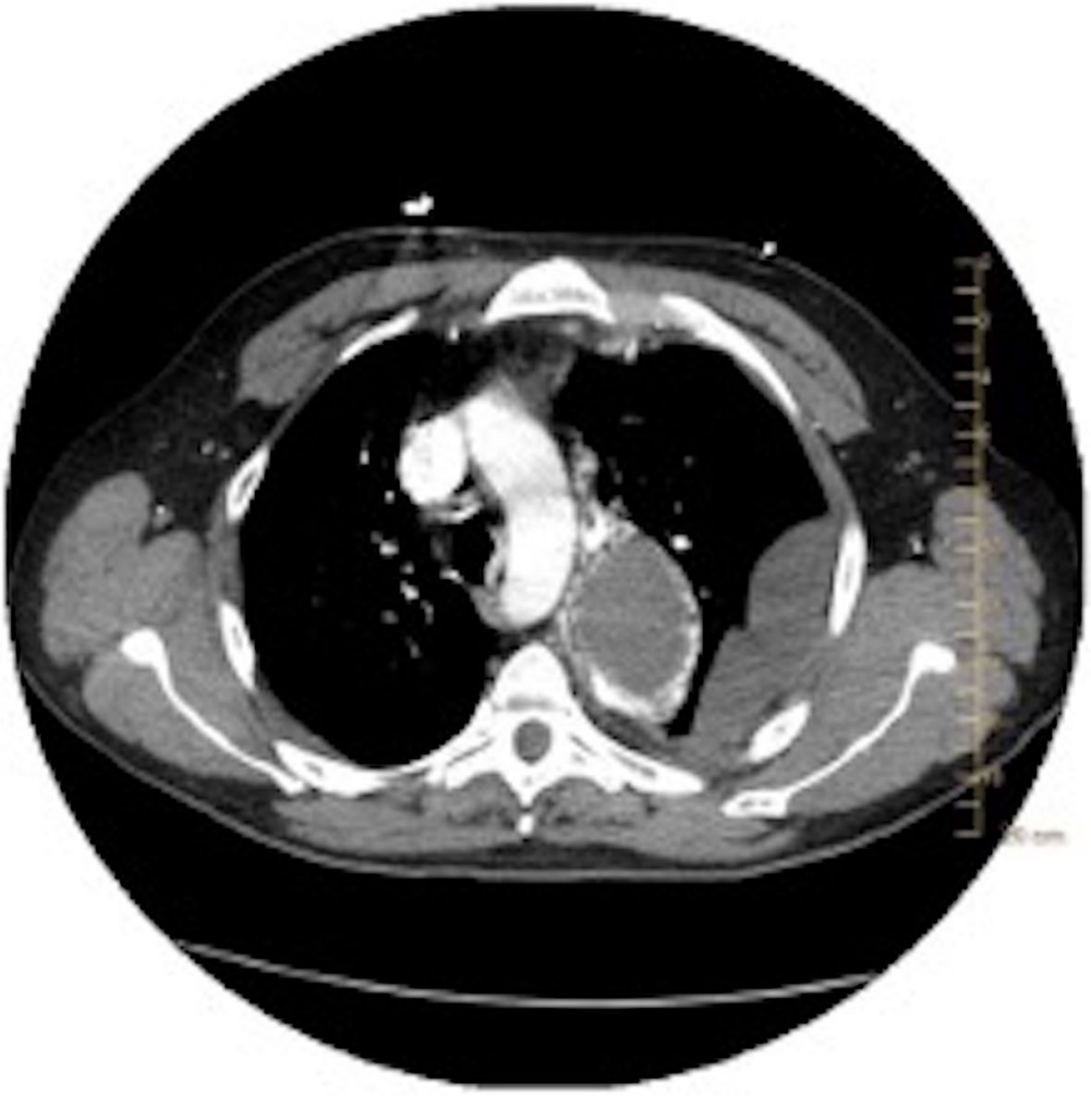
Axial image of CT chest with contrast demonstrating the posteromedial left upper lobe loculated fluid collection, concerning for an intralobular pulmonary sequestration
